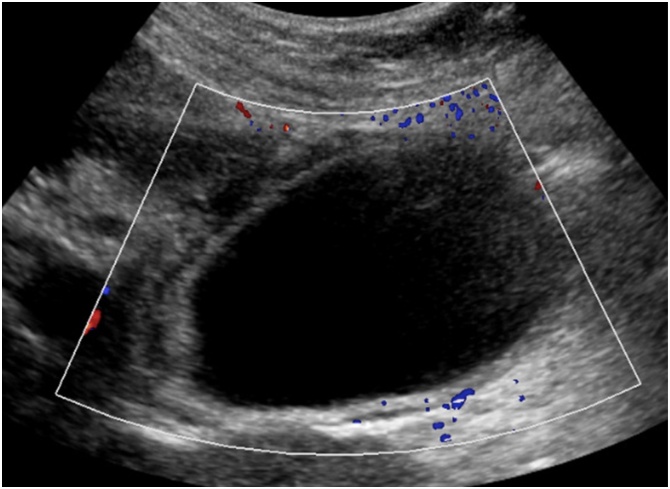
Ultrasound of a gallbladder in a 77-year-old female demonstrating 3 mm of wall thickness, distention, mild pericholecystic fluid, and no cholelithiasis. Intraoperatively, she was discovered to have a gallbladder volvulus.
